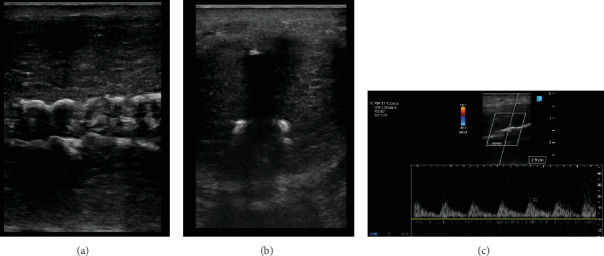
Penile ultrasonography. (a) Sagittal imaging with severe cavernosal calcifications. (b) Transverse imaging revealing shadowing from Peyronie's plaque and cavernosal calcifications. (c) Duplex ultrasonography showing poor peak systolic velocity of 17.10 cm/s.
